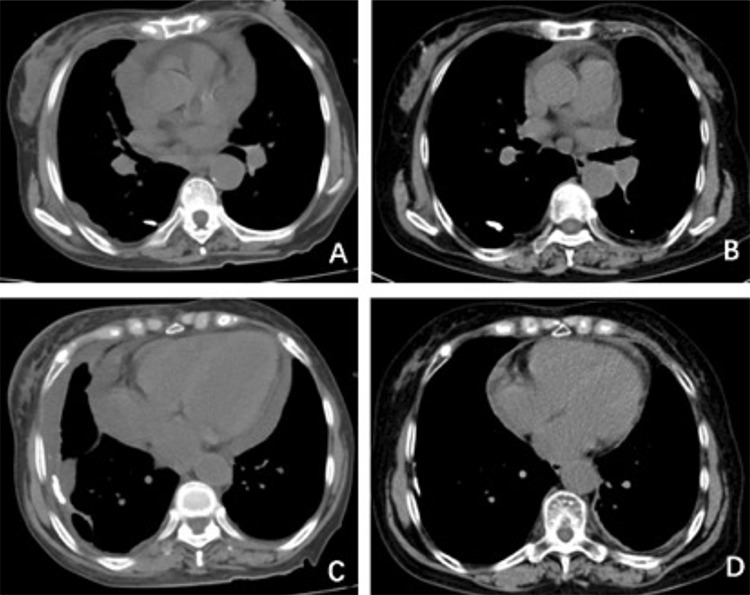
Computed tomography (CT) scans showed multiple enlarged lymph nodes in the mediastinum and pericardial and pleural effusion before treatment (A, C). CT scan showed complete disappearance of enlarged lymph nodes and pericardial or pleural effusion after treatment (B, D).
